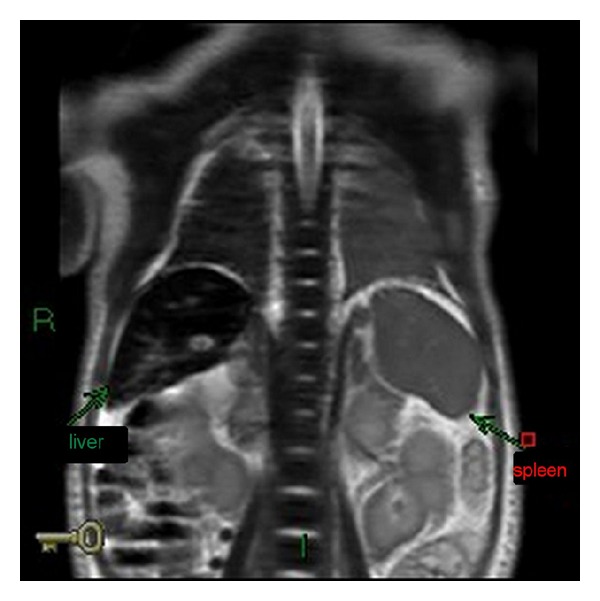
Abdominal MRI, coronal view, on day five of life showing low signal intensity of the liver parenchyma with preservation of the signal intensity of splenic parenchyma (T2WI protocol). Calculations of T2∗ showed rapid decay consistent with iron overload.
